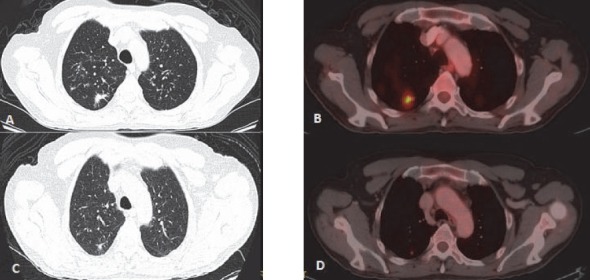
Lymphoma involving lung – Nodular lesion. Primary NHL of small intestine (not in picture). (A) Staging PET-CT scan shows soft tissue parenchymal nodule with irregular margin in right lung. (B) The lesion appears FDG avid. (C) Post 3 cycle chemotherapy shows anatomical reduction of the size of the lesion. (D) Complete metabolic response is seen in post therapy scan
